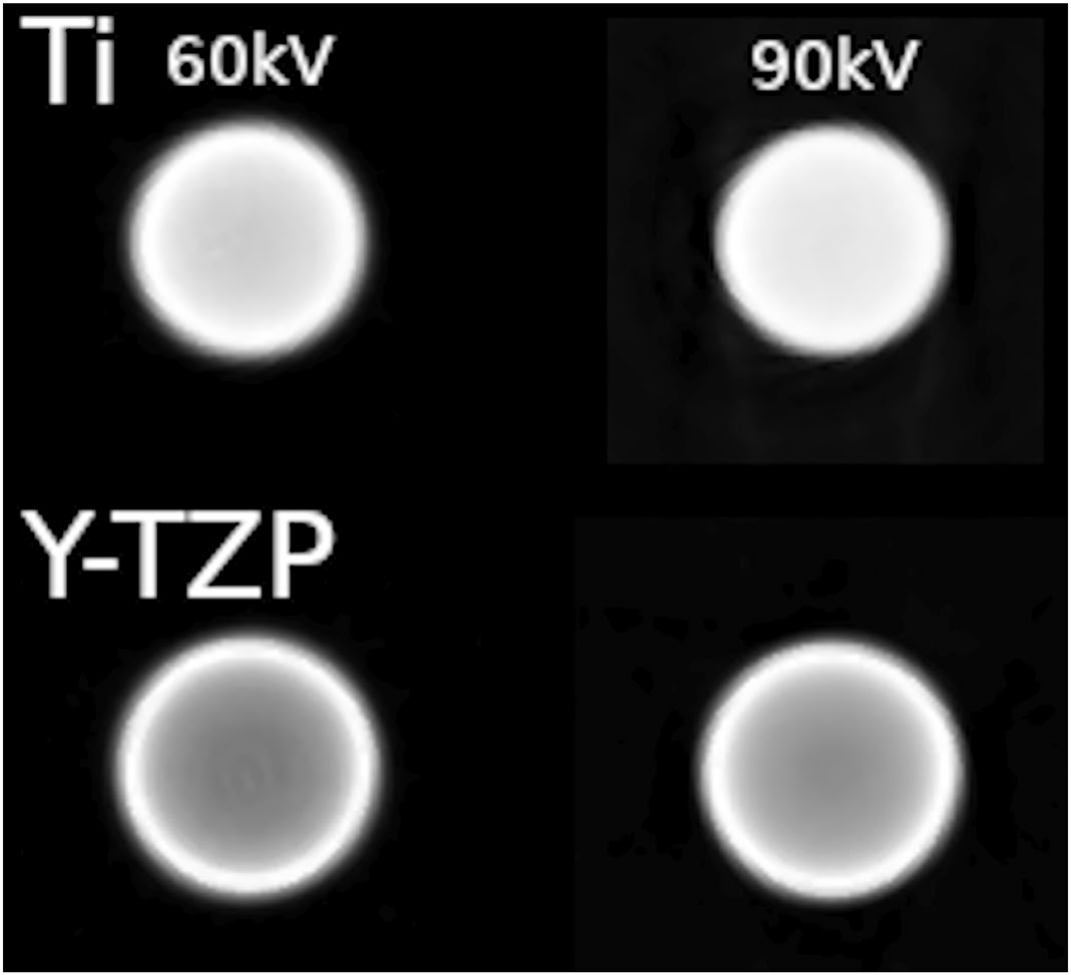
Slices through the DICOM CBCT-reconstruction of the implant samples for both materials and minimum (60 kVp) versus maximum (90 kVp) beam energy. Beam hardening is clearly visible in the center of the homogenouos implant as significantly darker gray values. This is particularly emphasized in the Y-TZP reconstructions (lower row).
